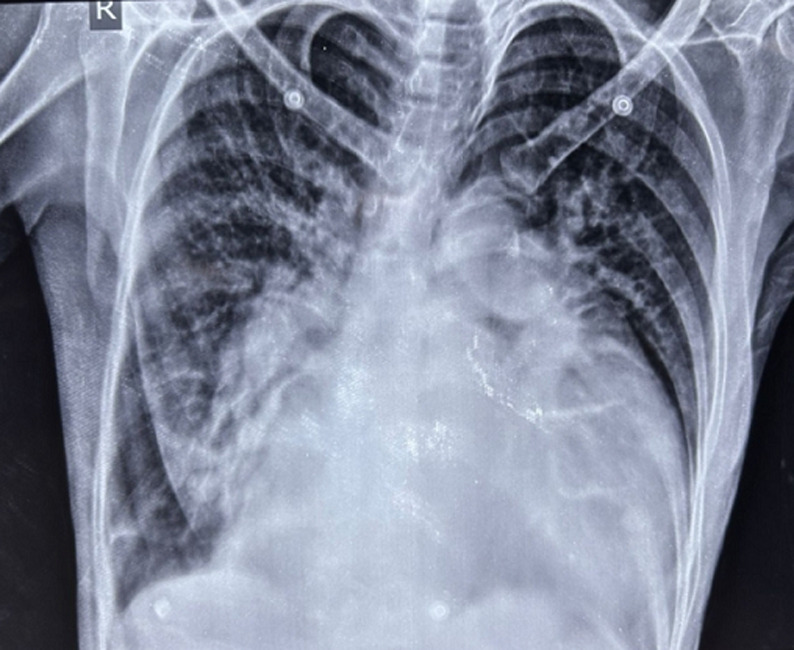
chest X-ray postero-anterior (PA) view demonstrating gross cardiomegaly in a patient with rheumatic heart disease
